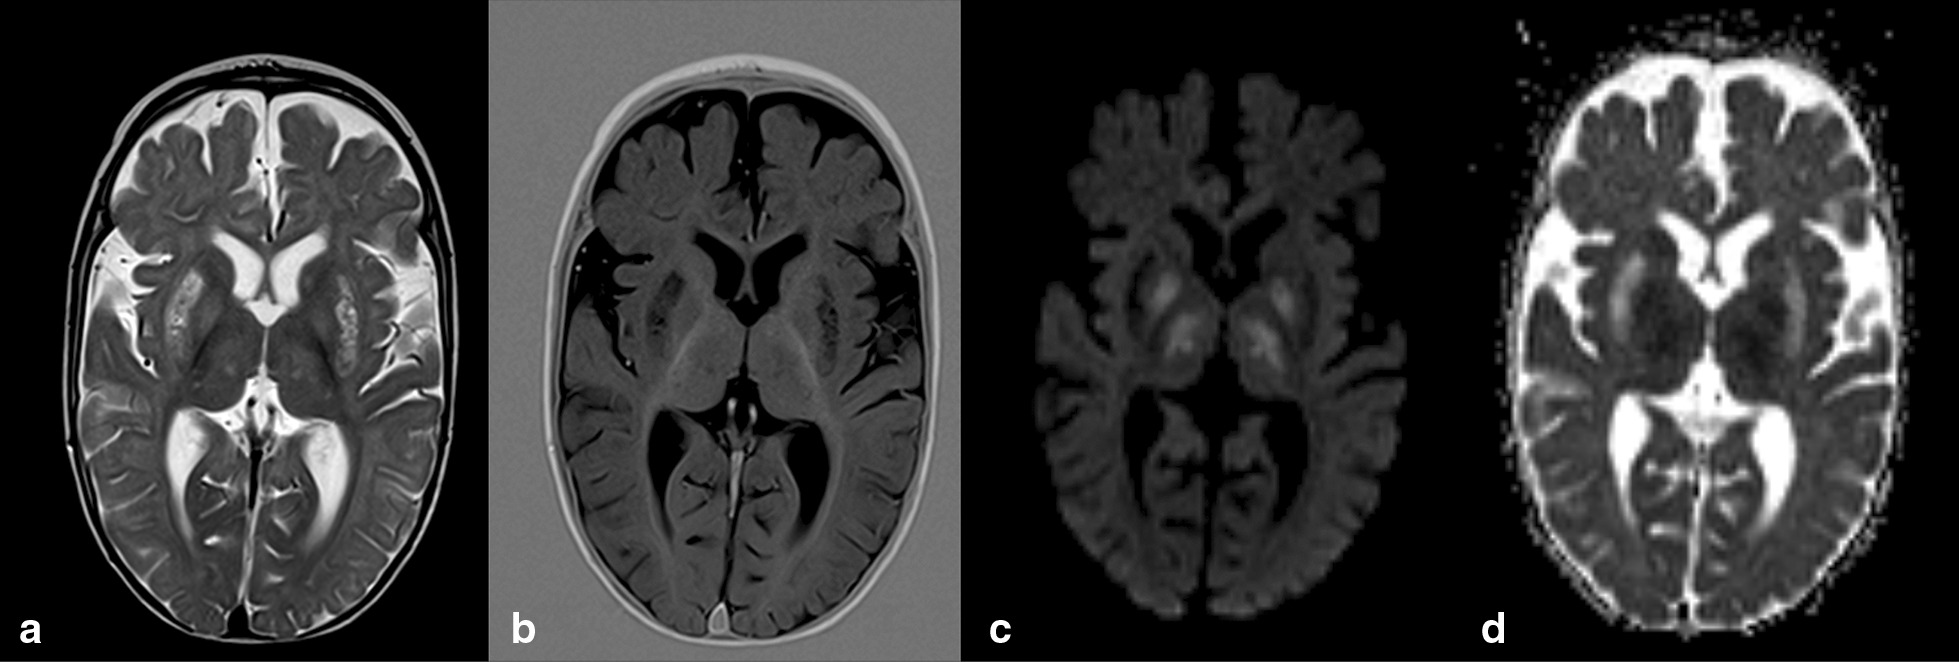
Magnetic resonance images of brain lesions of an 8 month old patient carrying a *PDHA1* mutation. Hyperintense bilateral and symmetrical lesions in the thalami, globus pallidus and putamina, on T2 weighted images, hypointense on T1 and with diffusion restriction suggestive of acute lesions; there is no diffusion restriction suggestive of chronic lesions. a axial T2, b axial T1 IR, c axial DWI, d axial ADC
